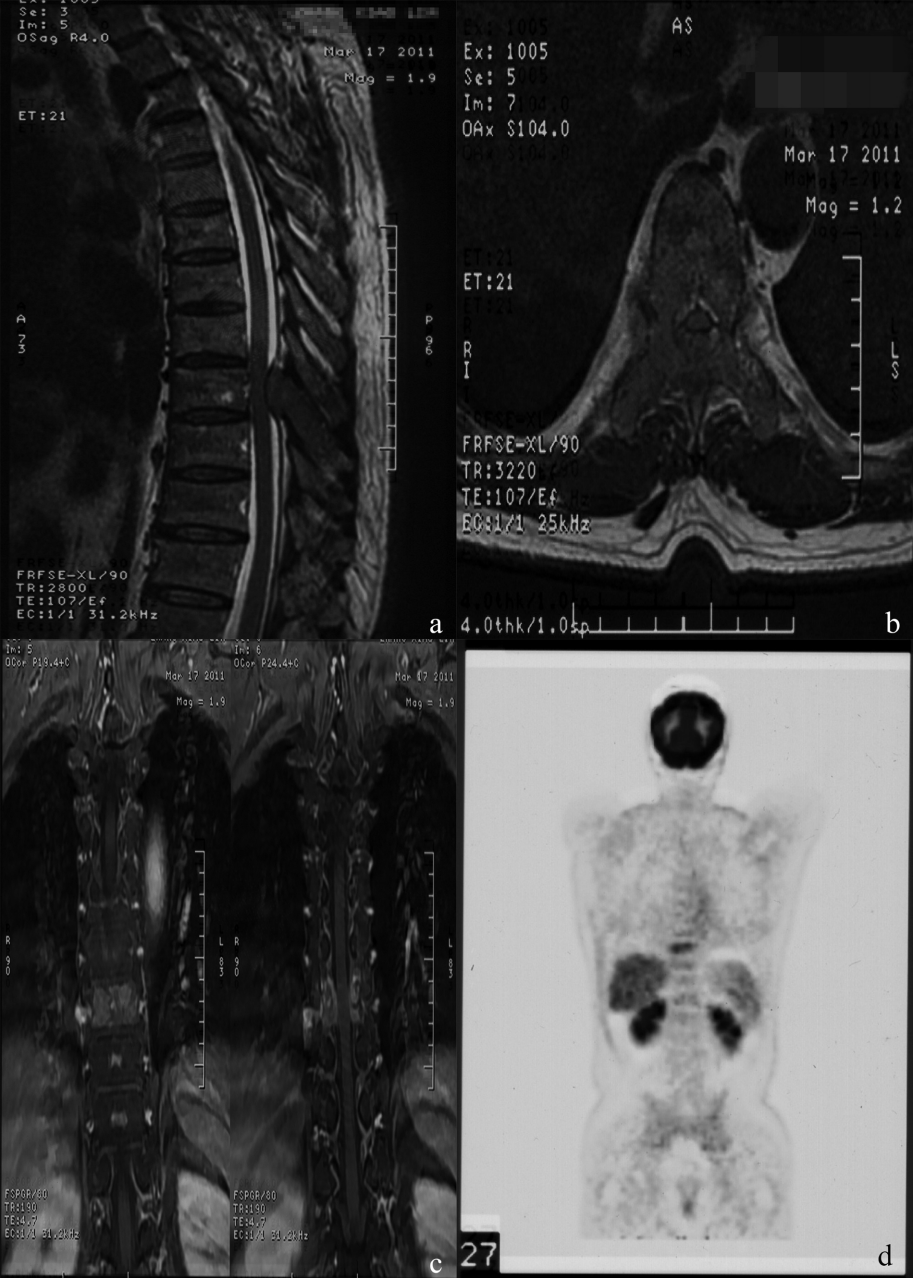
A.-D. Typical MRI and PET-CT images, showing abnormal signal of T9 body and appendix with spinal canal stenosis and spinal cord compression, abnormal ^18^F-fluorocholine uptake of the thoracic vertebrae.
